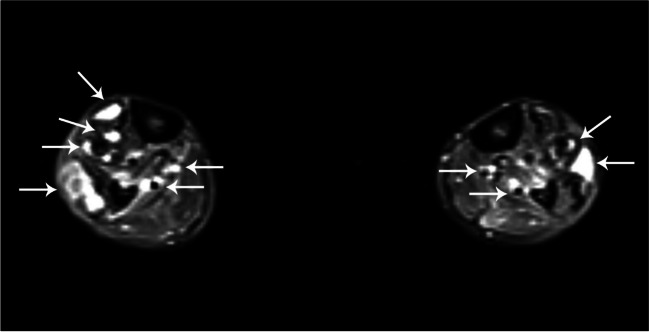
Sixty-three-year-old man with HIV and disseminated cryptococcus. Axial STIR MR image demonstrates multifocal soft tissues abscesses of the bilateral lower legs (*arrows*)
